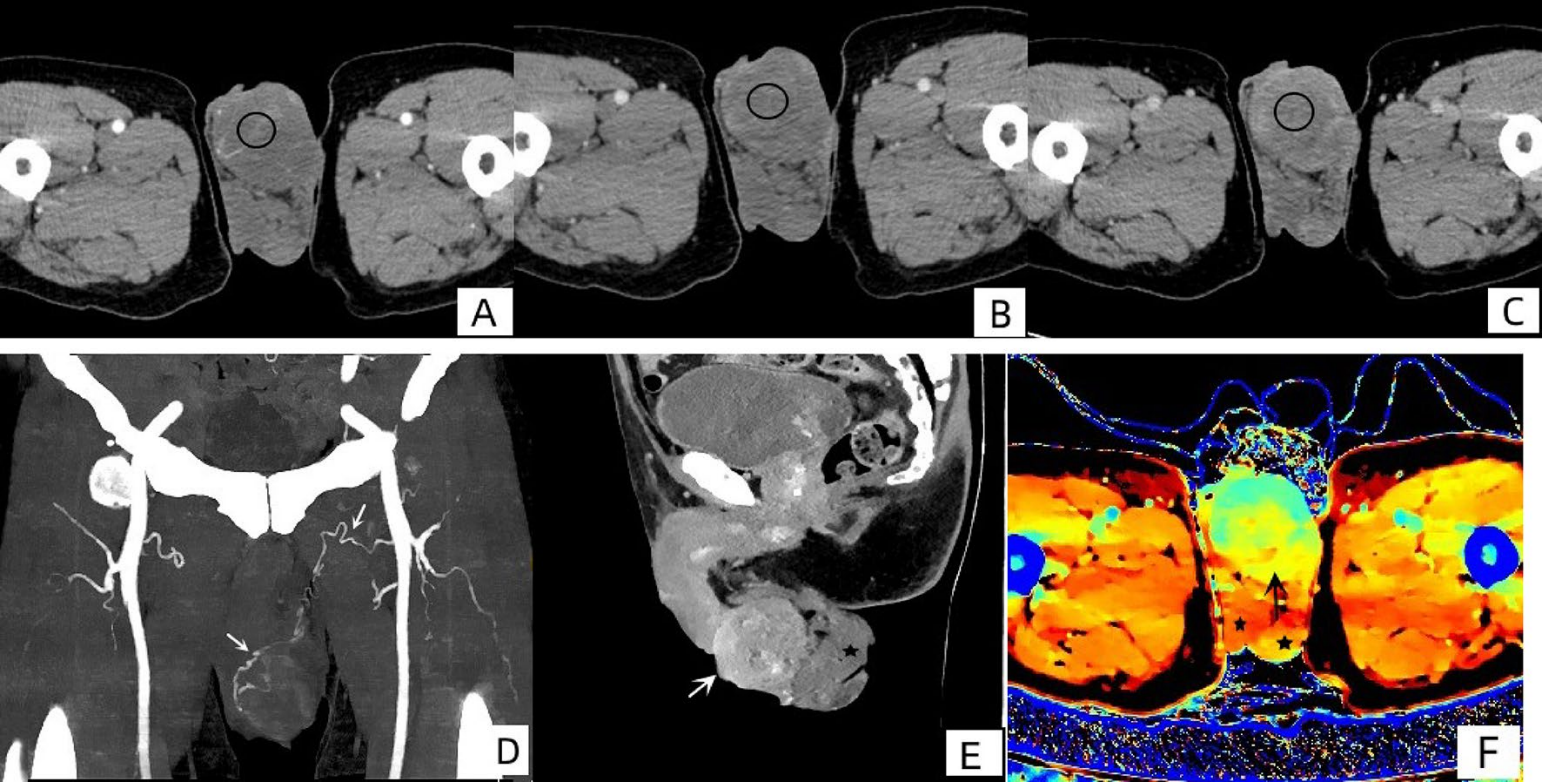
Dynamic enhanced scanning was performed in three phases, and the CT values of the mass during arterial phase (A), venous phase (B) and delayed phase (C) were 15, 33 and 48 HU, respectively. D A supply artery (white arrow) originating from the left femoral artery. E Delayed phase reconstruction shows uneven enhancement of the lesion (white arrow), with the left testicle (black star) in the background. F Z-effective image shows the mass (black arrow) and the testicle behind it (black star)
